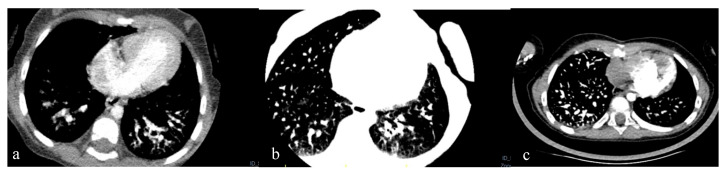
Images of lung parenchymal abnormalities in cases using CTA. (a) Centric nodules and ground-glass opacity in the lung parenchyma of a patient diagnosed with TA-VA concordance. (b) Sequelae lesions and emphysematous changes in the lung parenchyma of a patient diagnosed with DILV-VA discordance. (c) Air trapping in the lung parenchyma of a patient diagnosed with DILV-DORV.
